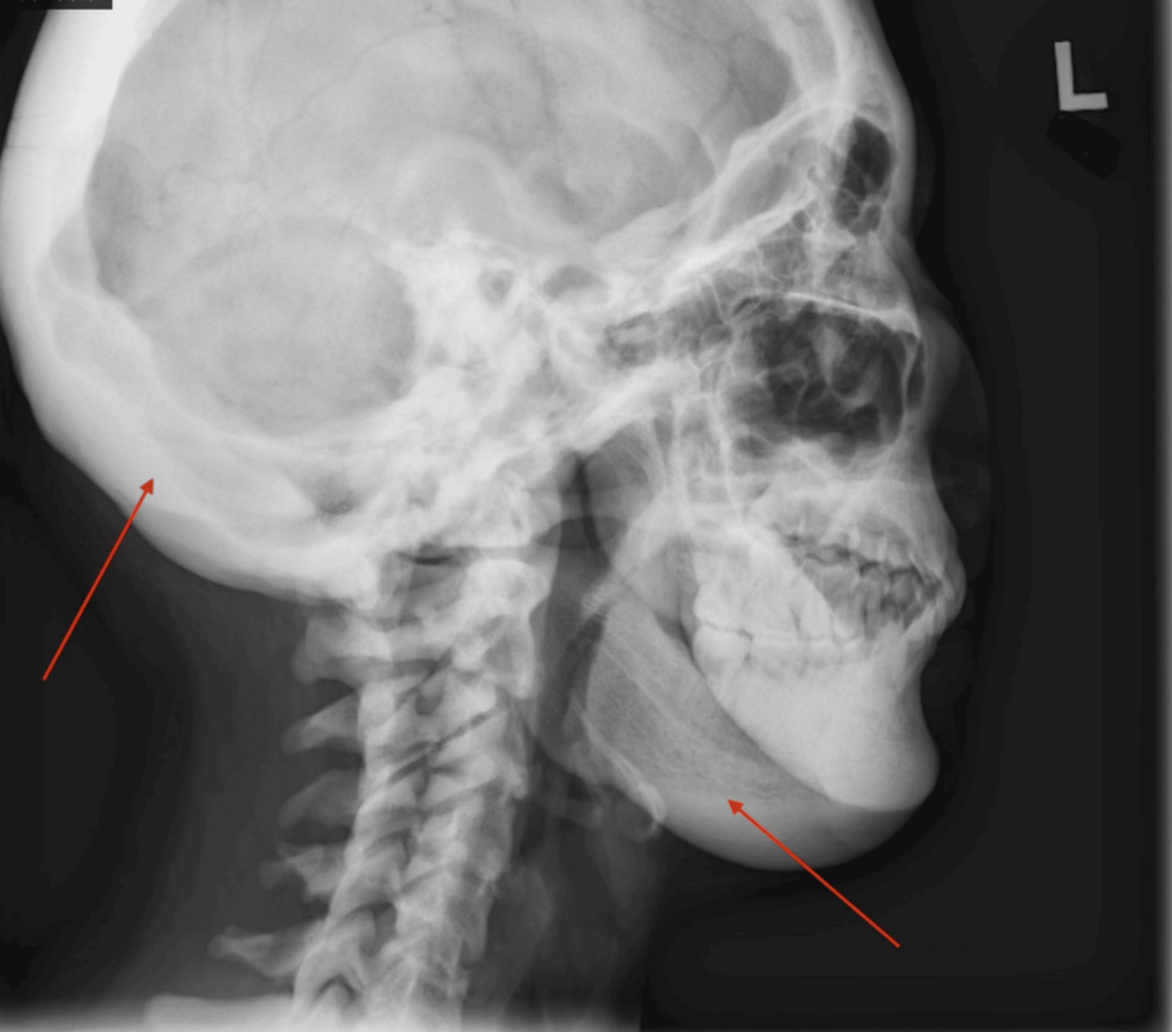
Lateral left X-ray of the mandible Increased bone mineral density of the mandibular body and symphysis and the skull
